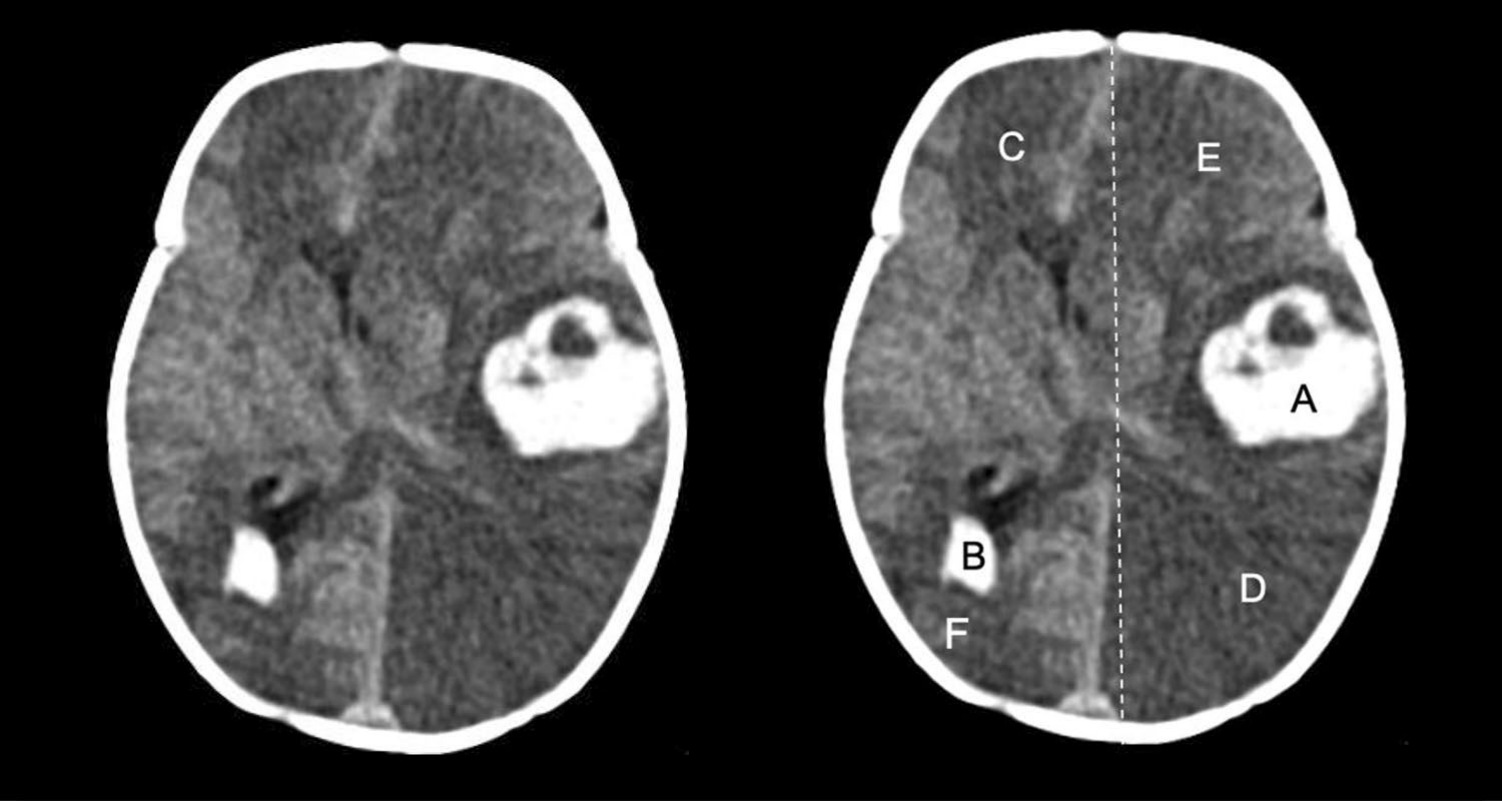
CT-scan showing a neonatal patient who developed a large intracerebral hematoma (A) with intraventricular extension (B), significant mass effect (mid-line depicted as a dotted line), and multiple ischemic lesion (C–F) during ECMO treatment. The original image is shown on the left.
